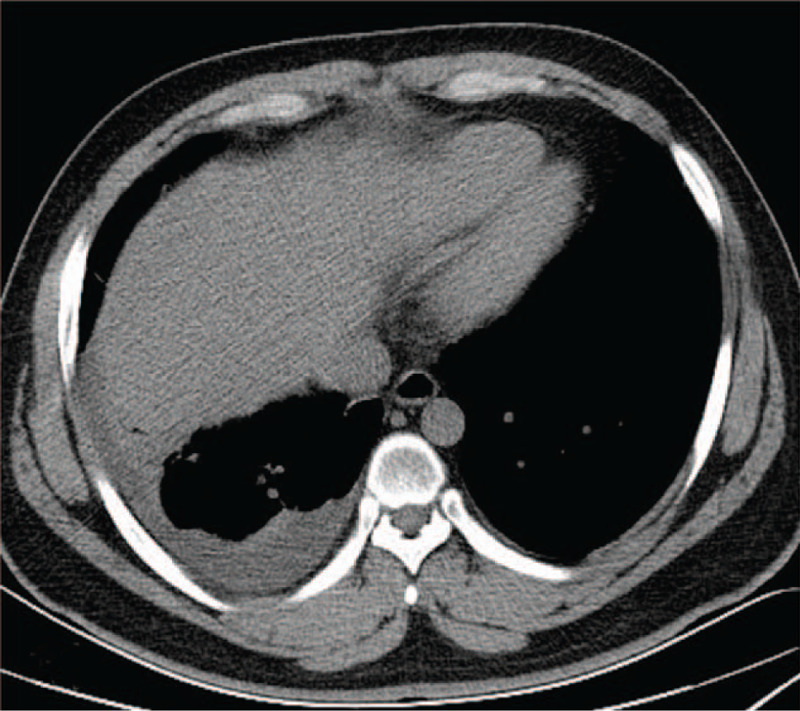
Chest CT on June 13 showed the right pleural effusion.
